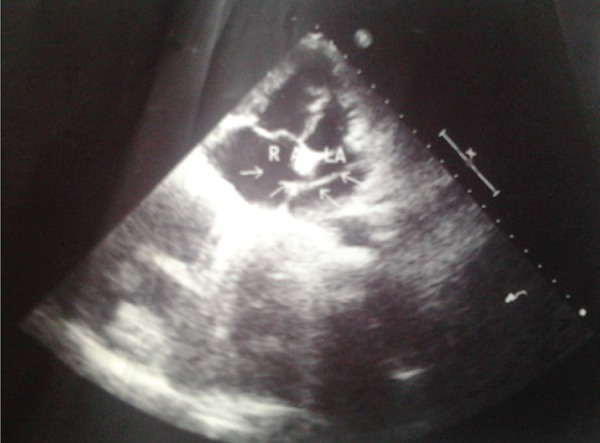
Echocardiogram Showing the Catheter Traversing the Interatrial Septum Abbreviations: RA, Right Atrium; LA, Left Atrium
